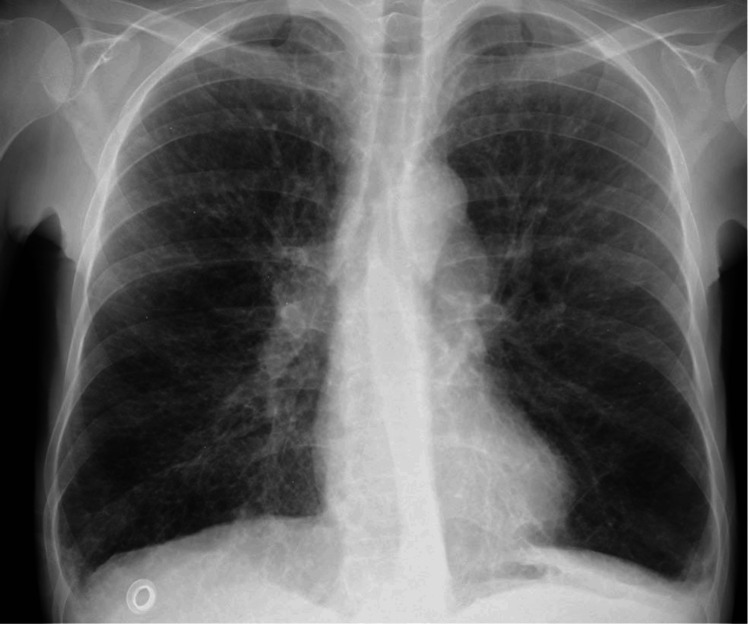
PA chest X-ray showing the diaphragm contours were flattened and there were an increase in bilateral bronchovascular scarring and ventilation in the basal areas. The PA chest radiograph shows bilateral diaphragmatic flattening, increased bronchovascular markings suggestive of bronchial wall thickening or scarring, and signs of hyperinflation with enhanced basal ventilation. PA: posterior-anterior
